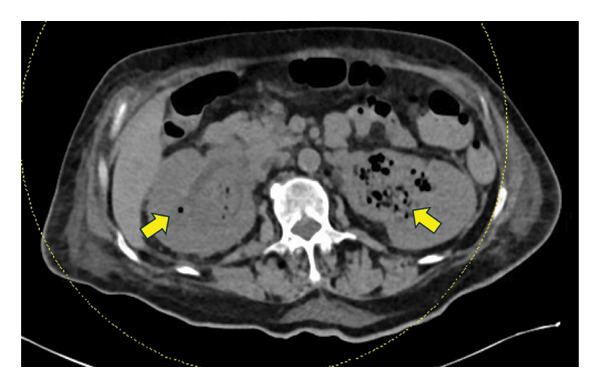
Noncontrast computed tomography (CT) abdomen and pelvis axial view, demonstrating severe dilation of the left and right collecting systems with fluid and gas as indicated by yellow arrows.
